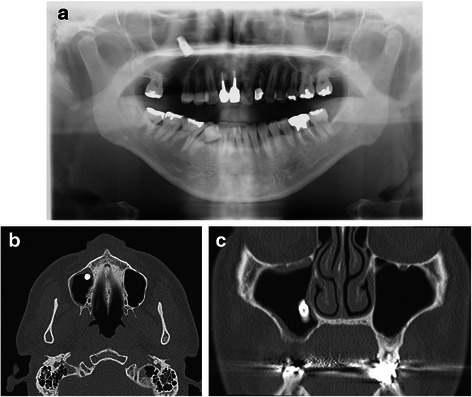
a Panoramic radiograph. b, c Computed tomography images obtained prior to the operation (b, axial section; c, coronal section)
